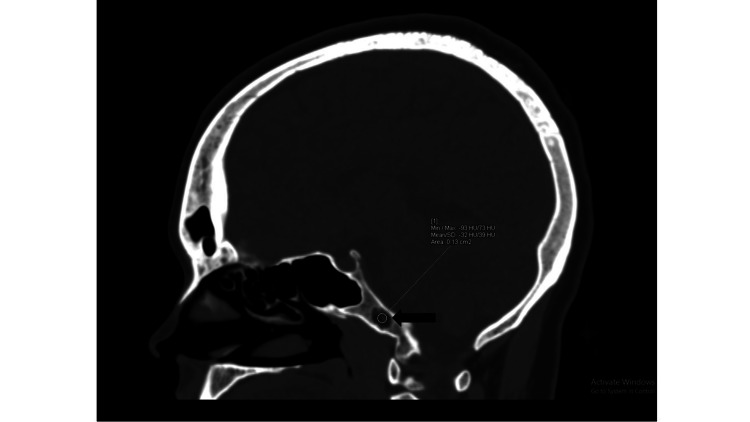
NCCT image of the skull (sagittal section) demonstrating intraosseous lipoma at the clivus (black arrow) with ROI placement revealing fatty attenuation of the lesion. NCCT, non-contrast computed tomography; ROI, region of interest
